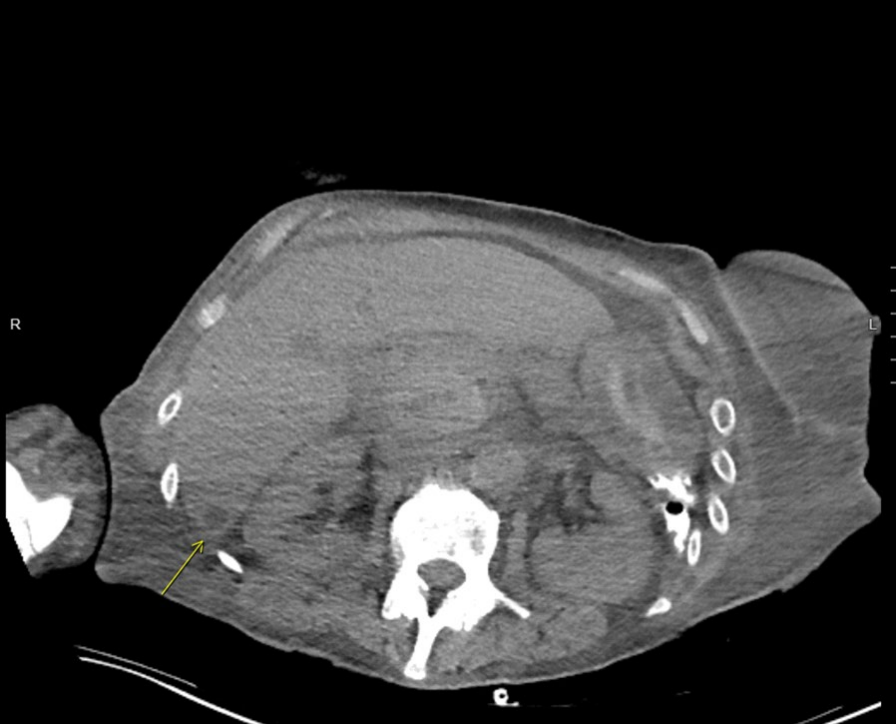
CT chest, abdomen, pelvis indicating the hypoattenuating liver nodule
